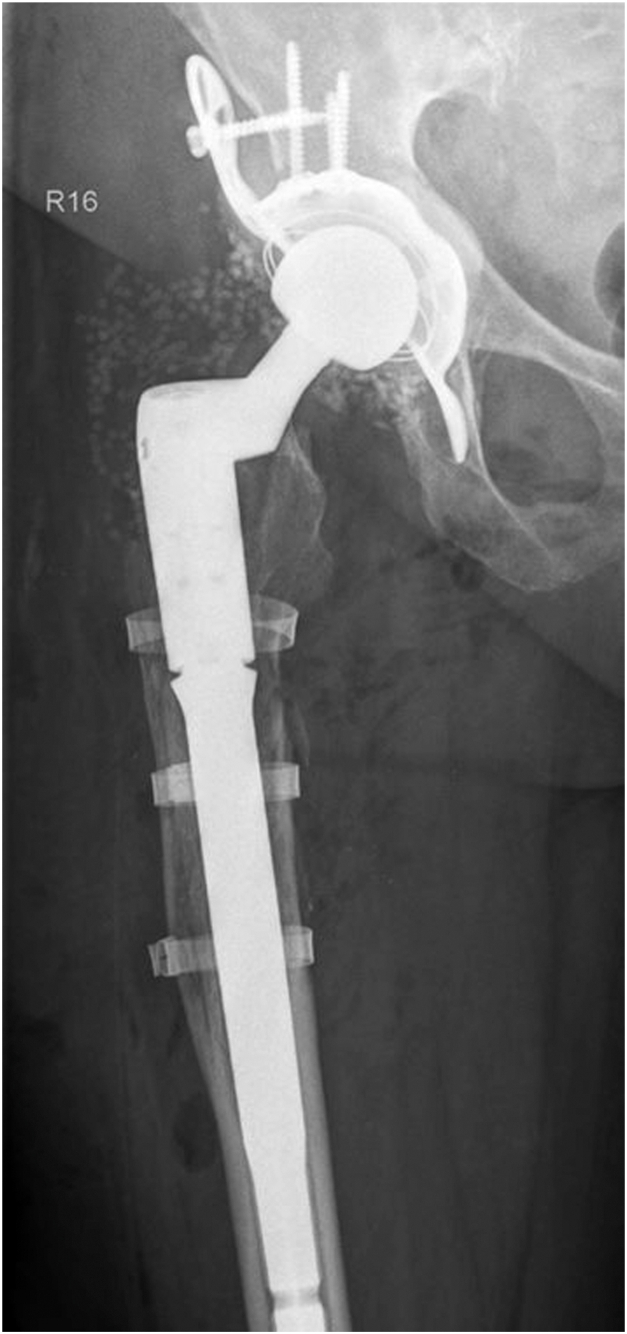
Postoperative anteroposterior radiograph of the right hip, postoperative imaging showcases the Burch-Schneider Ring, cemented polyethylene cup and modular cementless stem (Implants: Burch-Schneider Revision 56 mm, Fa. Zimmer, Warsaw, Indiana, US; Exeter X3 Rimfit 36/52 mm, Fa. Stryker, Kalamazoo, Michigan, US; Mutars modular stem 16/200 mm, middle piece 50 mm, head piece 135°/32 mm, Fa. Implantcast, Buxtehude, Germany; Biolox 36 mm ceramic head size XL, Fa. CeramTech, Plochingen, Germany).
